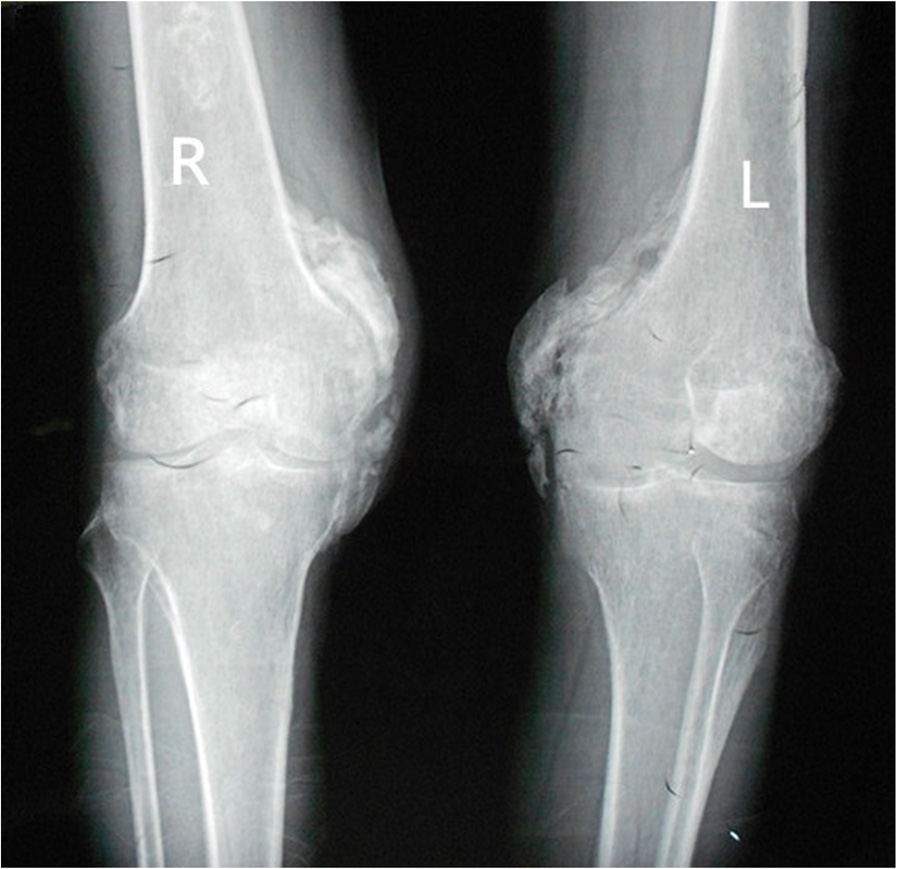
Preoperative radiographs of anterioposterior and lateral radiographs of knees shows HO on the peripheral areas of knee-joints, especially on the interior.
